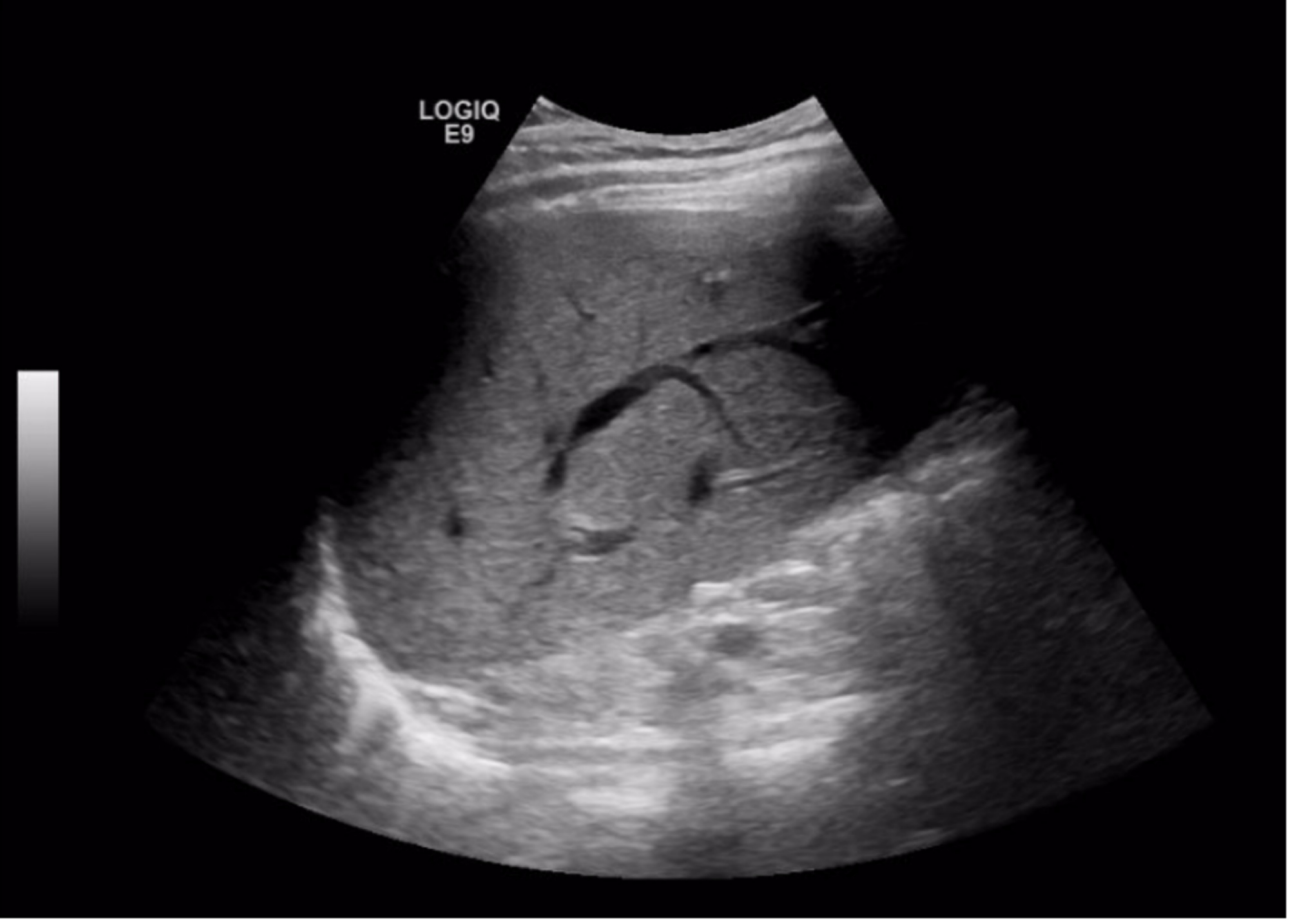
Ultrasound of the liver shows heterogenous liver texture
